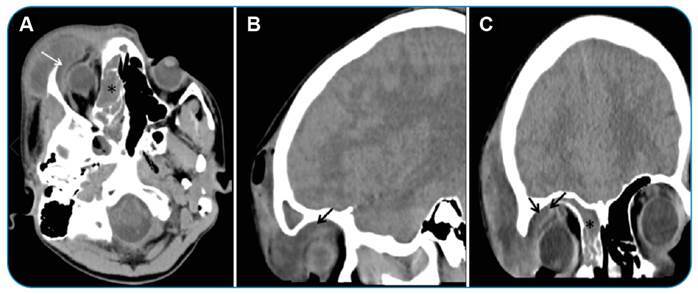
Axial (A), sagittal (B), and coronal (C) sectional images of the brain CT show the extension of the subperiosteal abscess into the orbit (arrows) and the density of ethmoid cells on the right side.
